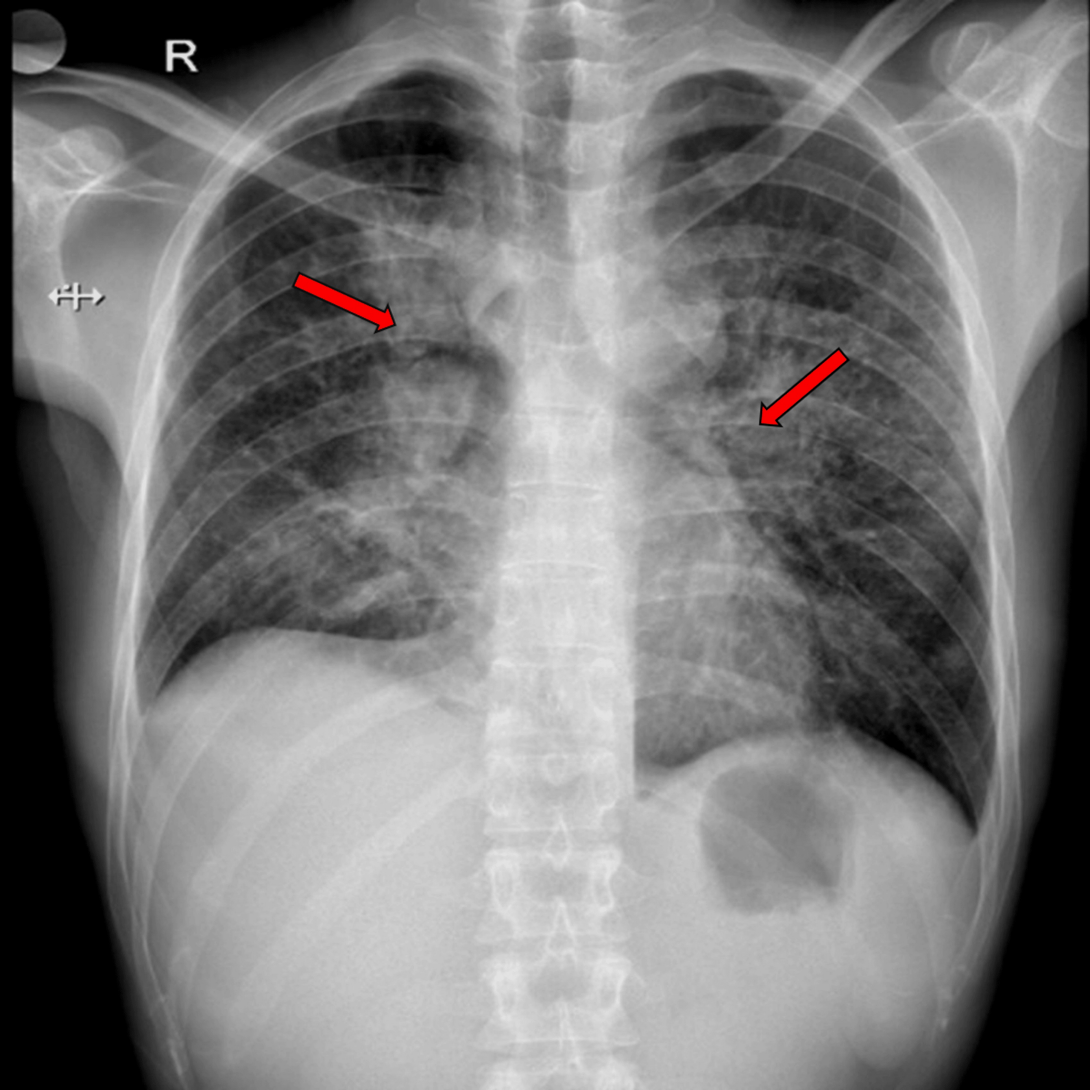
Plain chest radiograph on presentation showing bilateral hilar opacities (red arrows).
